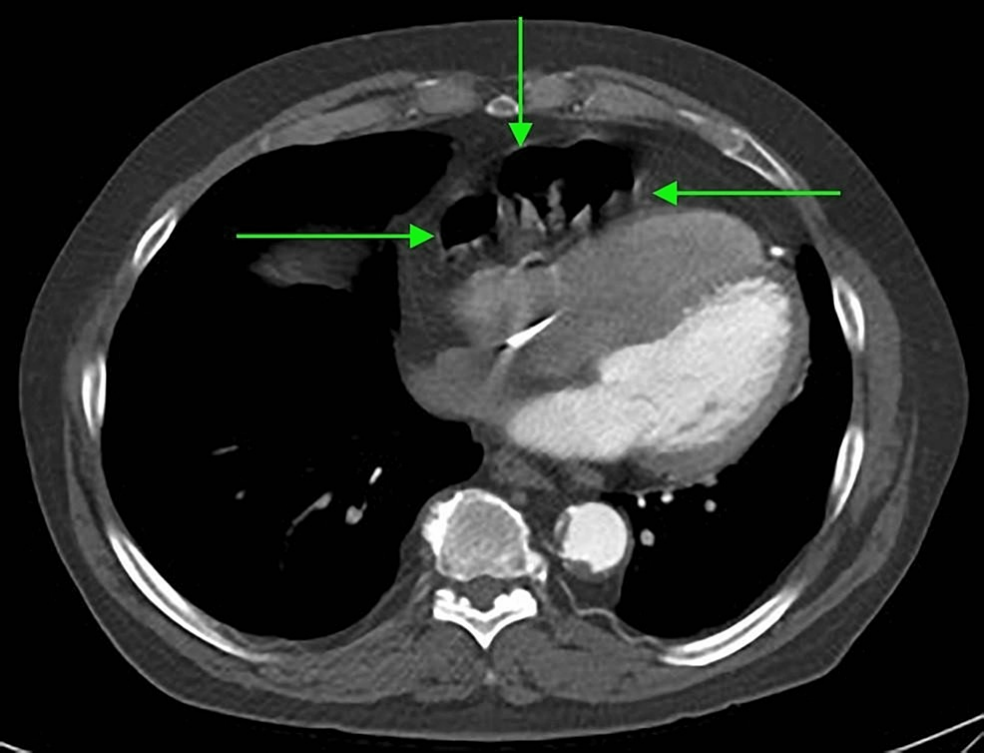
CTA of the chest, horizontal sections revealing diaphragmatic hernia CTA: computed tomography angiogram.
